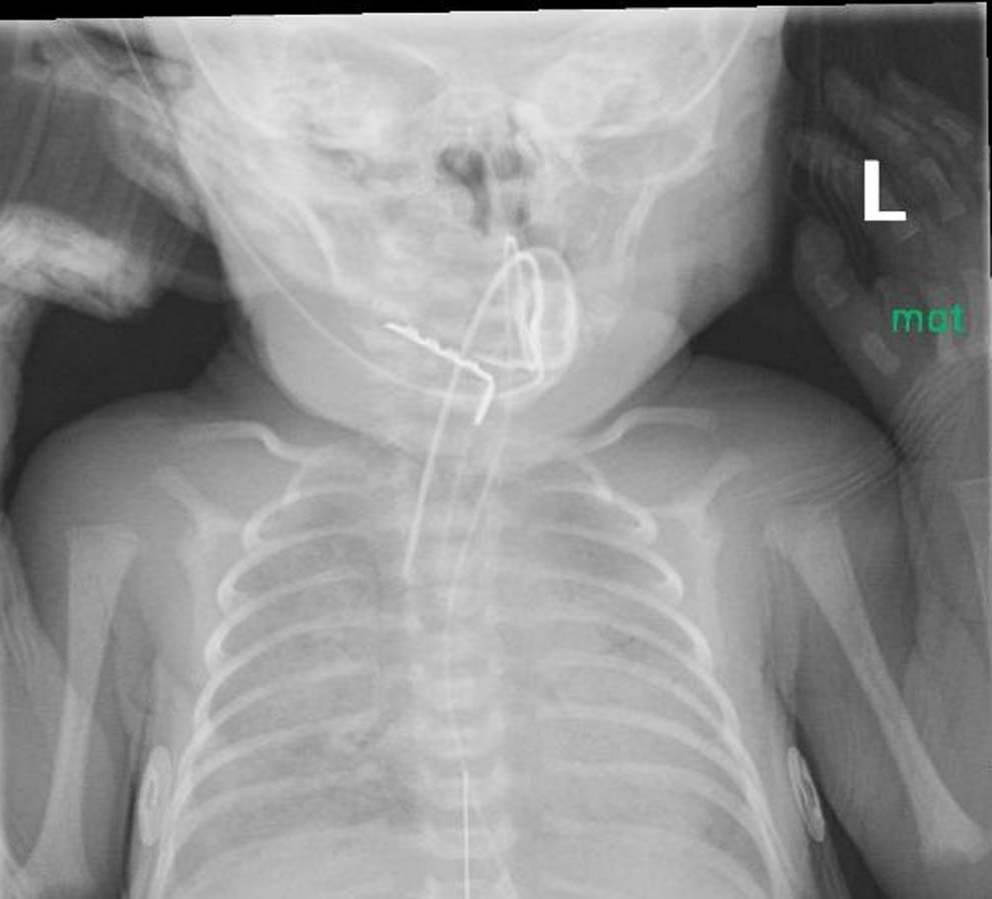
X-ray shows the presence of an L-shaped object in the oral cavity on the day of admission.
